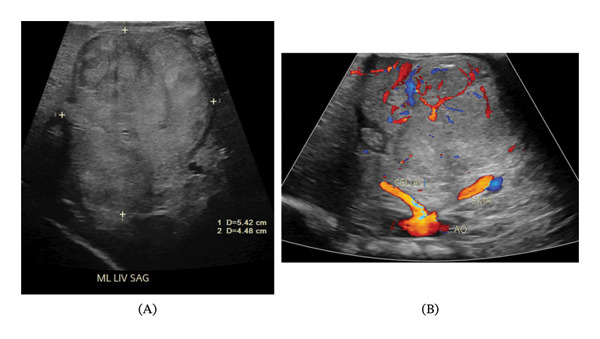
(A) Gray scale ultrasound images demonstrate a 5.4 × 4.5 cm heterogeneous solid mass centered in the mesentery of the abdomen and inferior to the pancreas. (B) Midline sagittal color ultrasound images demonstrate the mass is anterior to the aorta, celiac axis, and superior mesenteric artery. It is hypervascular and supplied by both the celiac artery and the superior mesenteric artery.
